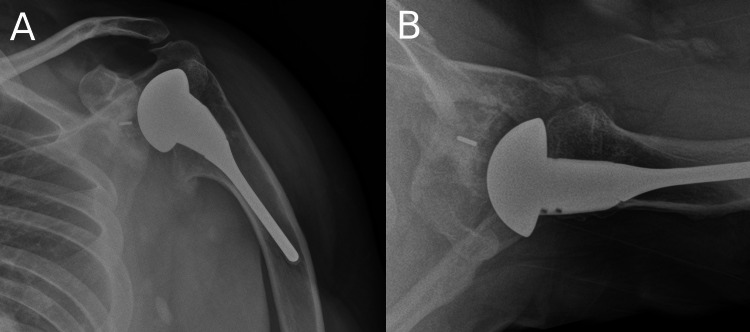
X-rays of the left shoulder displaying the revised implants at final check-up (A) Anteroposterior view; (B) Axial view
